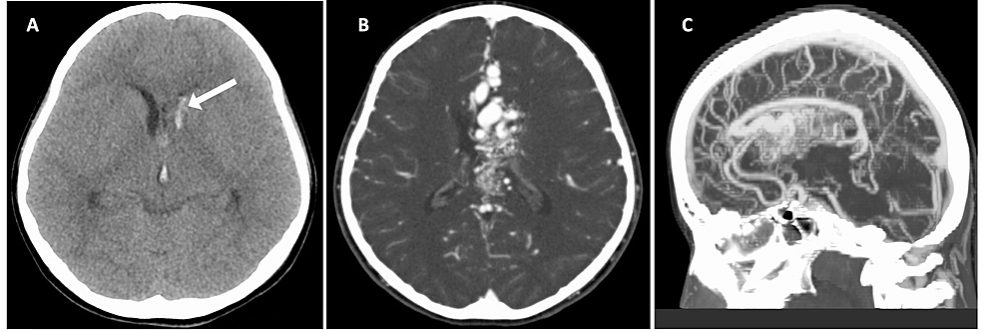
Preoperative CT (A) Preoperative CT shows the intraventricular hemorrhage centered in the left anterior horn (arrow).
